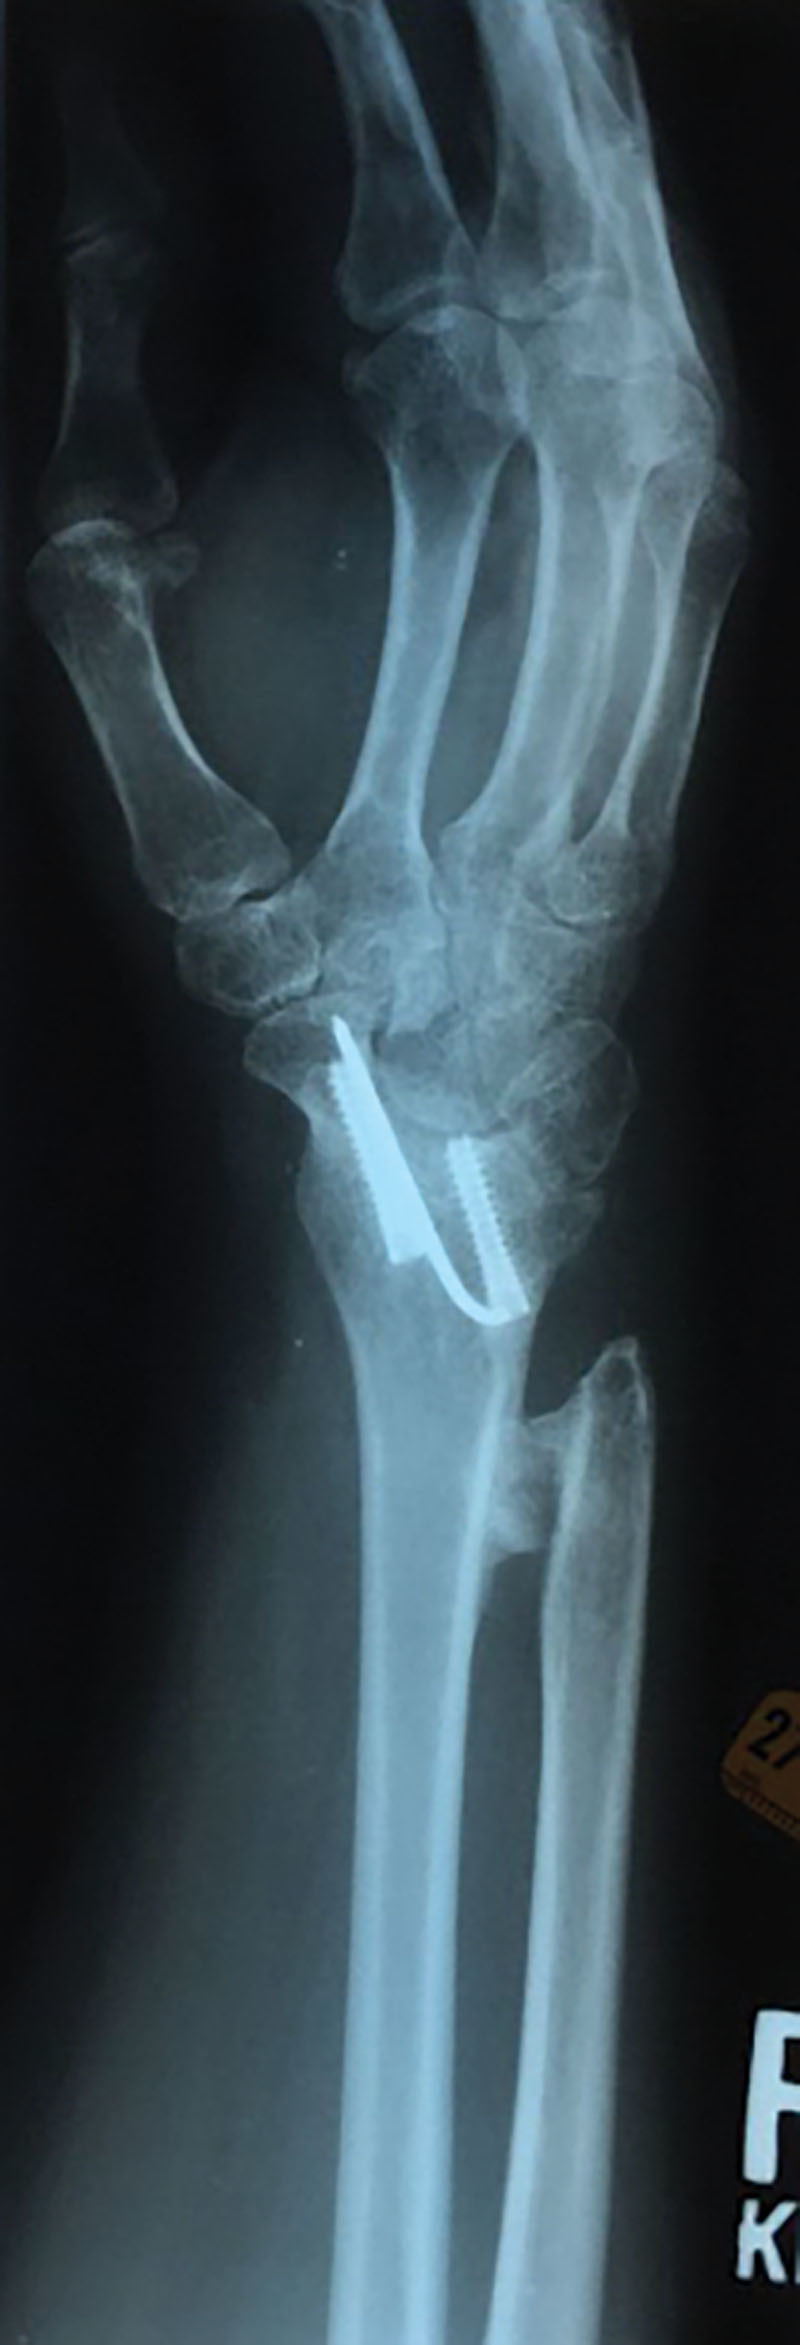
Patient 1 preoperative roentgenogram showing heterotopic ossification of the right distal radius and ulna.
